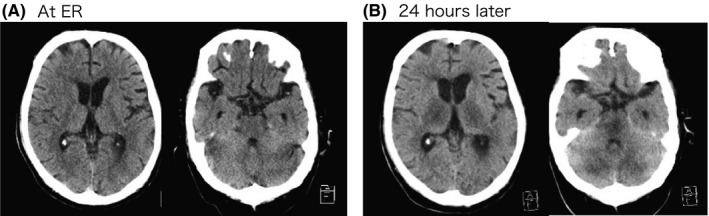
Head computed tomography at (A) emergency room (ER) and (B) 24 h later after the admission. The image at ER had been normal; however, those 24 h later showed low density at the bilateral thalamus, cerebellum, and brain stem.
